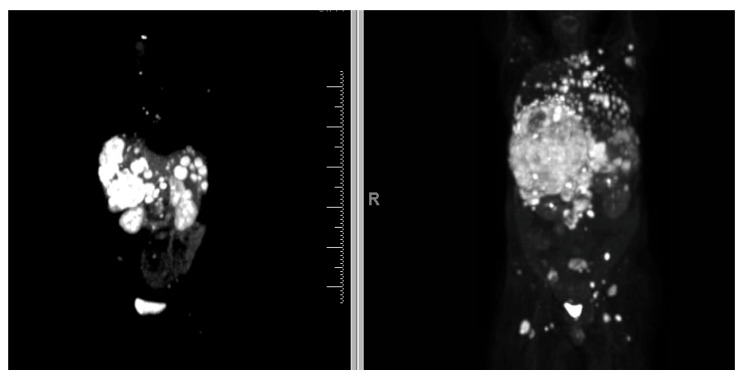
Comparative PET imaging in a patient with a G3 NET. (Left) [^68^Ga]Ga-DOTATATE PET with SSTR-positive hepatic metastases but SSTR-negative thoracic and retroperitoneal disease. (Right) [^18^F]FDG PET positive for metastatic hypermetabolic disease in retroperitoneum and thorax and relatively negative disease in liver. FDG = fluorodeoxyglucose; G = grade; NET = neuroendocrine tumor; PET = positron emission tomography; SSTR = somatostatin receptor.
